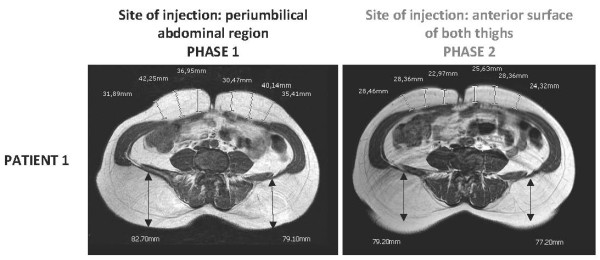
Abdominal MRI scans of patient 1 when injecting pegvisomant (10 mg/day) subcutaneously in her abdomen (Phase 1) and thighs (Phase 2).
